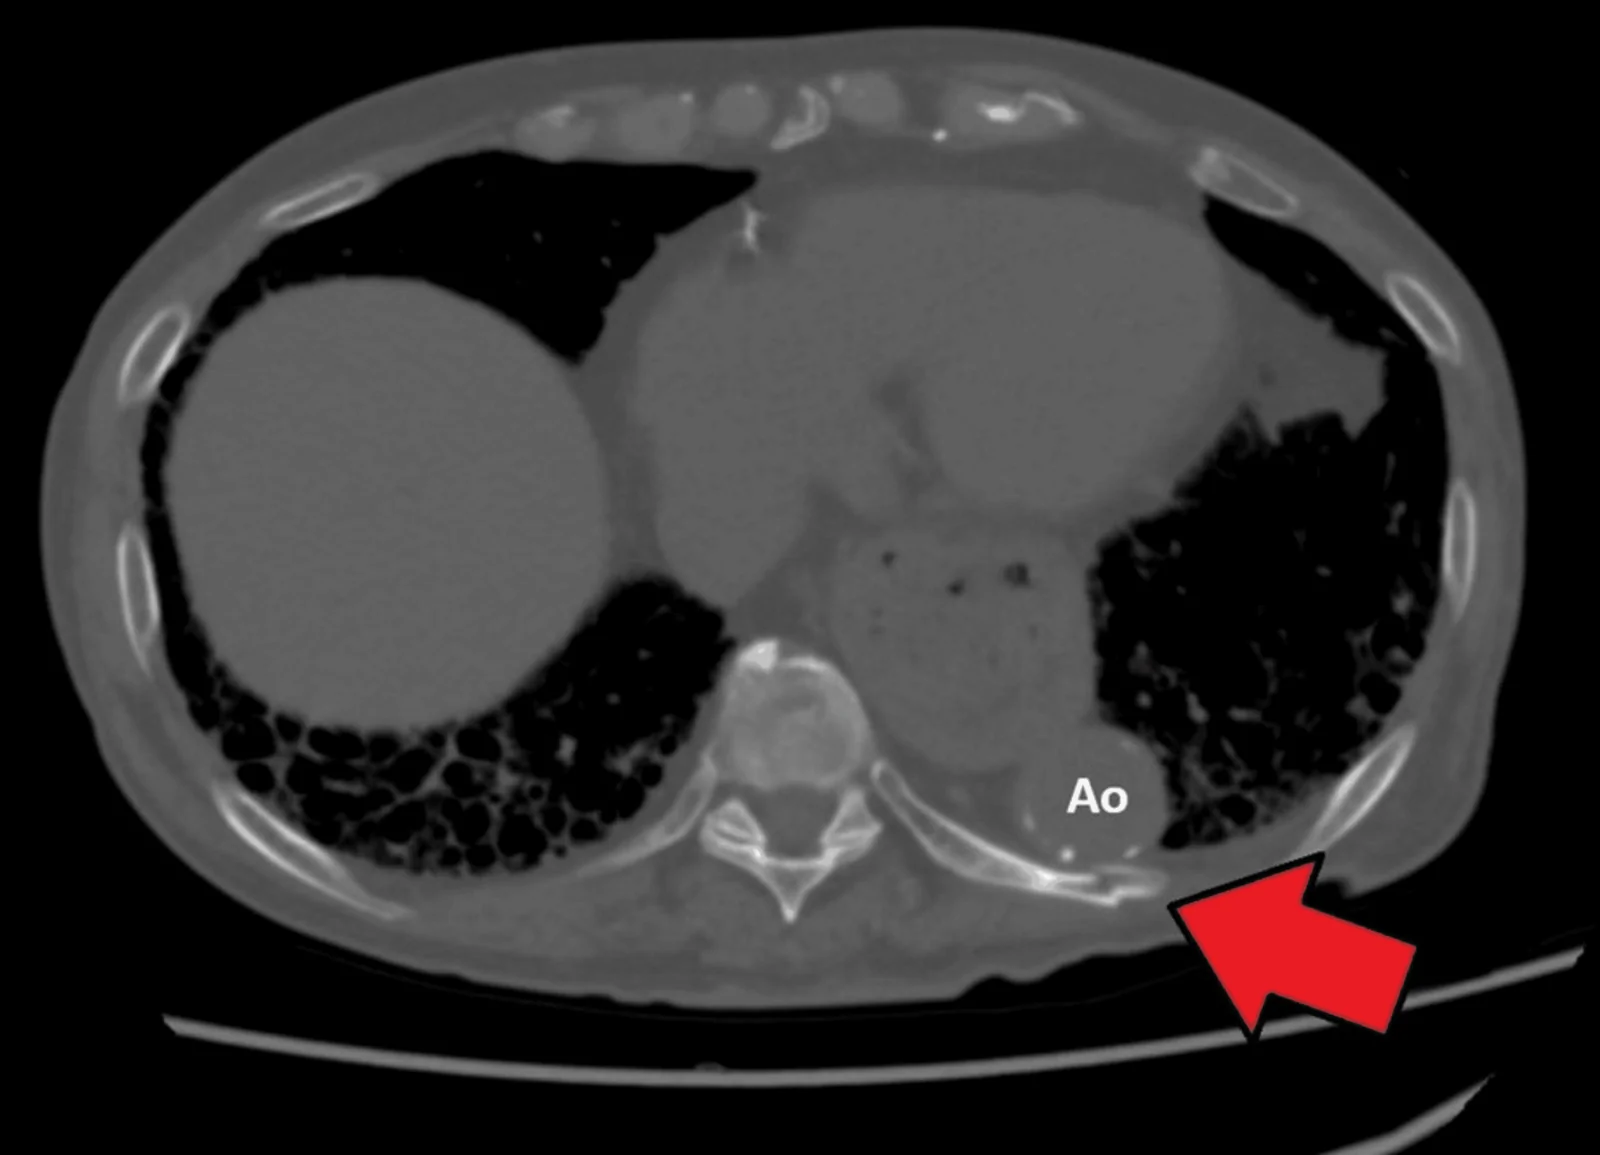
Chest computed tomography obtained after the initial fall. The left 10th rib fracture is evident, but no significant hemothorax indicative of aortic injury is present at this stage. The red arrow indicates the fractured rib, which is located in close proximity to the descending aorta (Ao).
